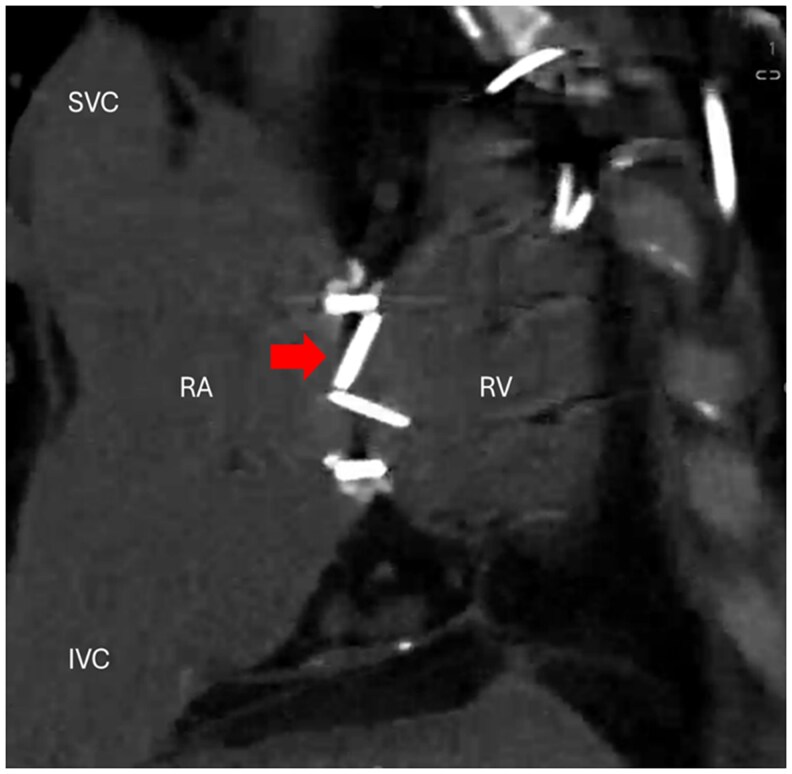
Non-contrast gated cardiac computed tomography showing the immobile anterior leaflet of the 27 mm St Jude bileaflet mechanical valve (arrow). SVC, superior vena cava; IVC, inferior vena cava; RA, right atrium; RV, right ventricle.
